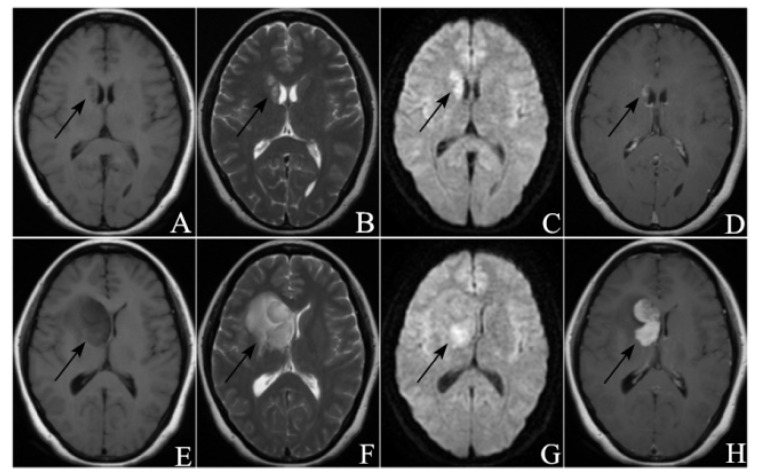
A sixty-seven-year-old male PCNSL patient didn’t receive consolidation WBRT after initial MTX chemotherapy, and the PFS1 was 11.2 months. KPS of this patient was 70 at first relapse. (A–D) were MRI images before HD-MTX rechallenge, and (E–H) were MRI images 60 days after treatment ((A,E) from T1WI, (B,F) from T2WI, (C,G) from DWI, (D,H) from CE-T1WI). The tumor was located in the right basal ganglia and showed homogeneous enhancement. After treatment, the tumor obviously enlarged and the edema aggravated, and this patient was judged as PD.
